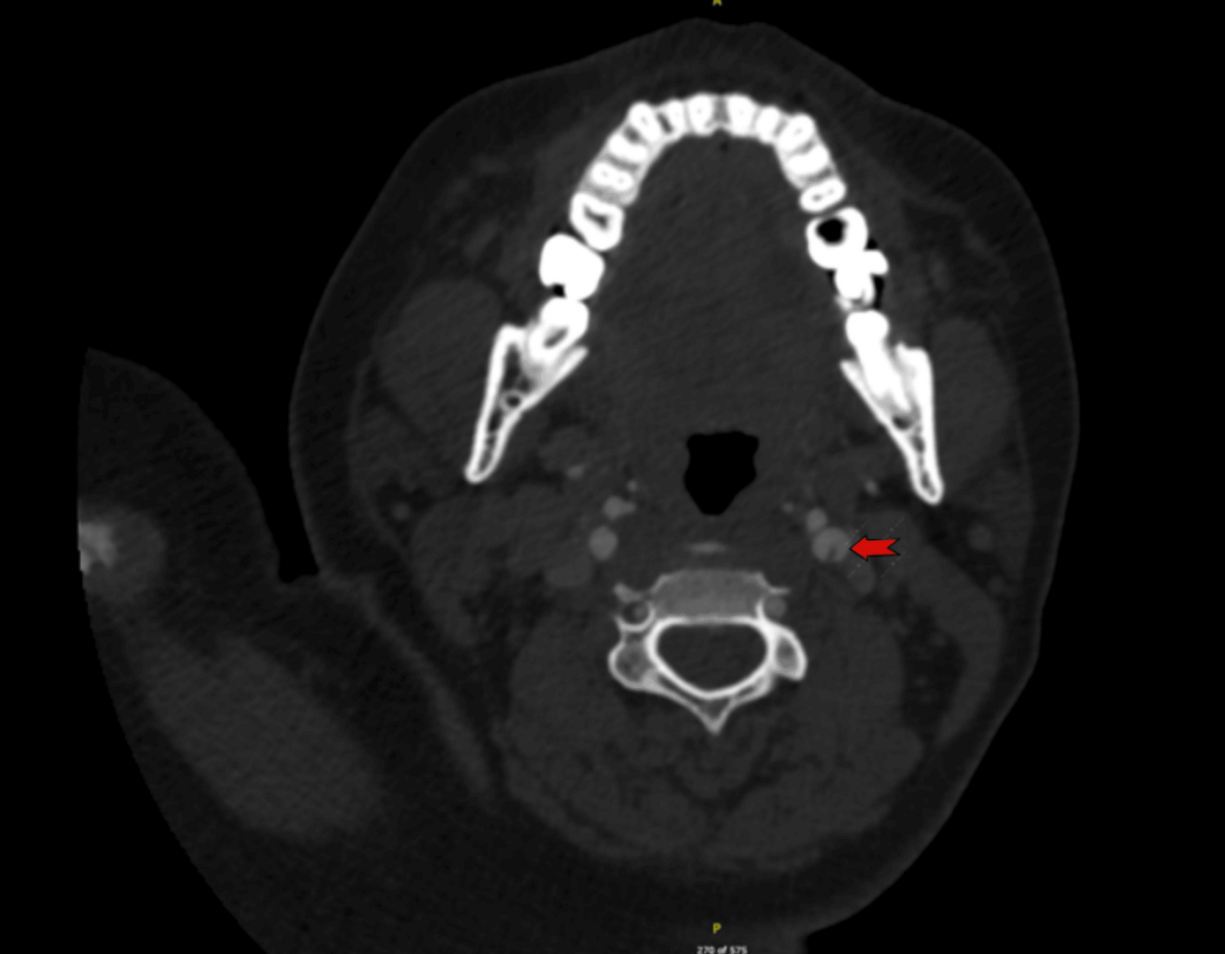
CT Angiography (Axial view) Red arrow showing irregular hypodensity at the left internal carotid artery origin, suggestive of a web or plaque.
